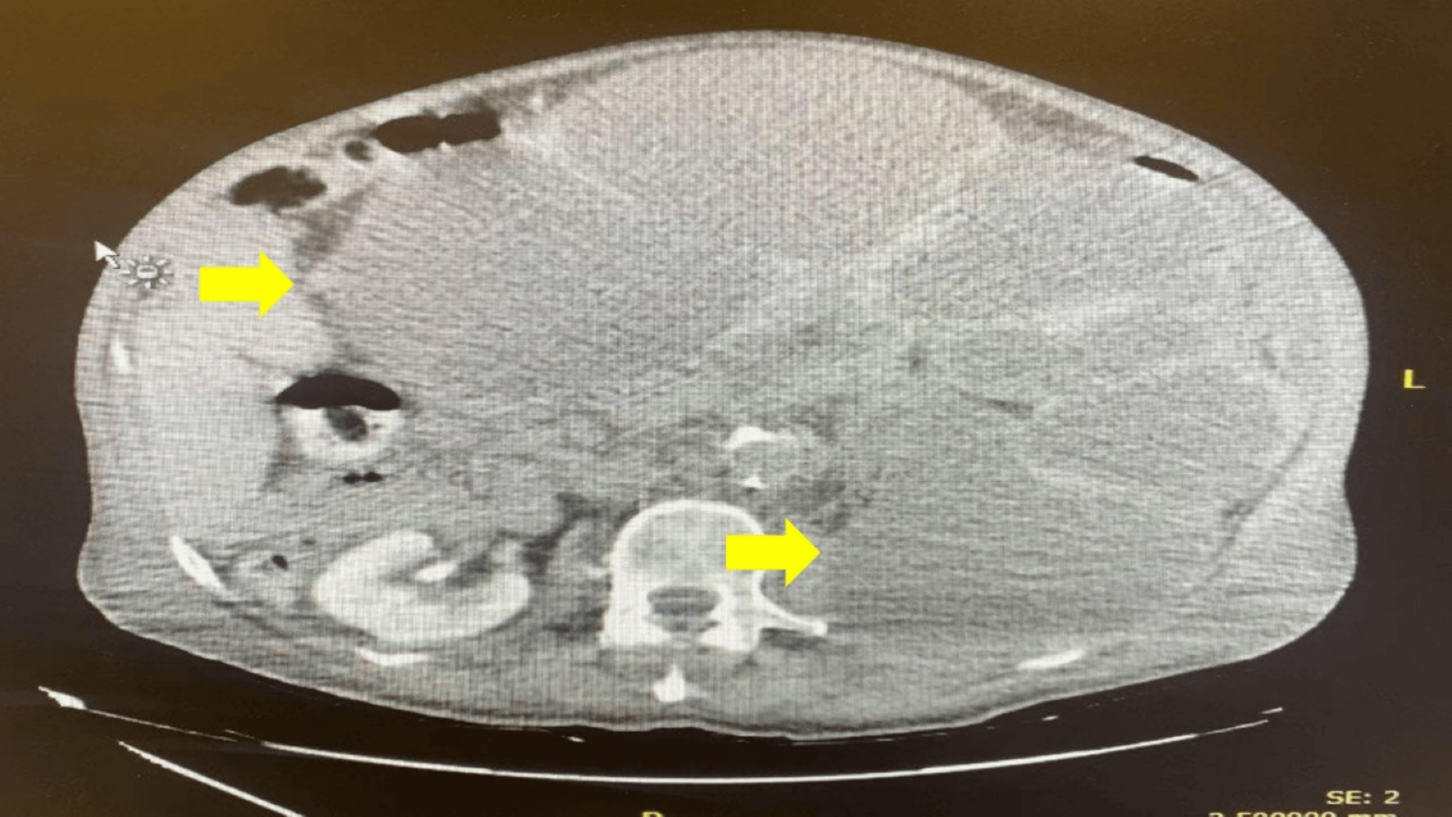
A CT scan of the abdomen and pelvis without IV contrast demonstrated a severely enlarged left kidney occupying the left side of the abdominal cavity. Radiological findings were suggestive of chronic ureteral obstruction complicated by severe xanthogranulomatous pyelonephritis of the left kidney (yellow arrows).
